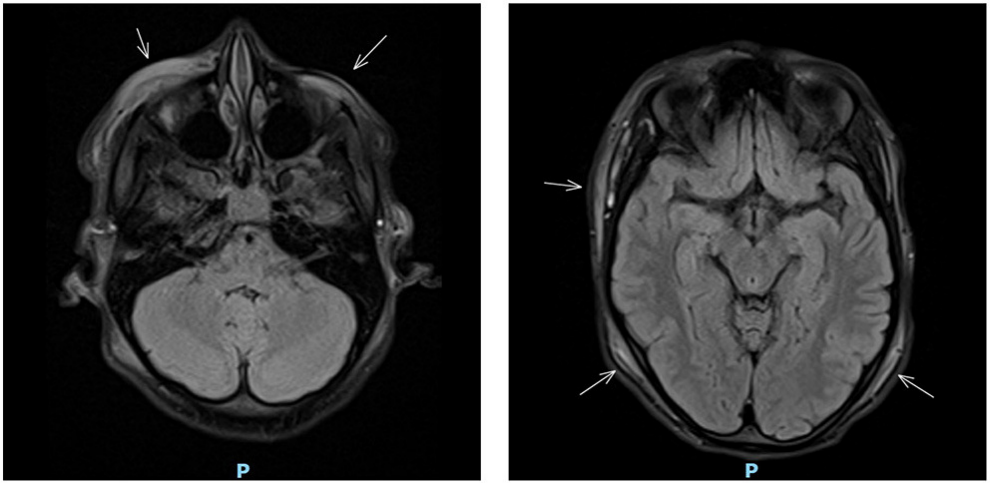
Cranial MRI showing diffuse subcutaneous edema (indicated by arrows).
